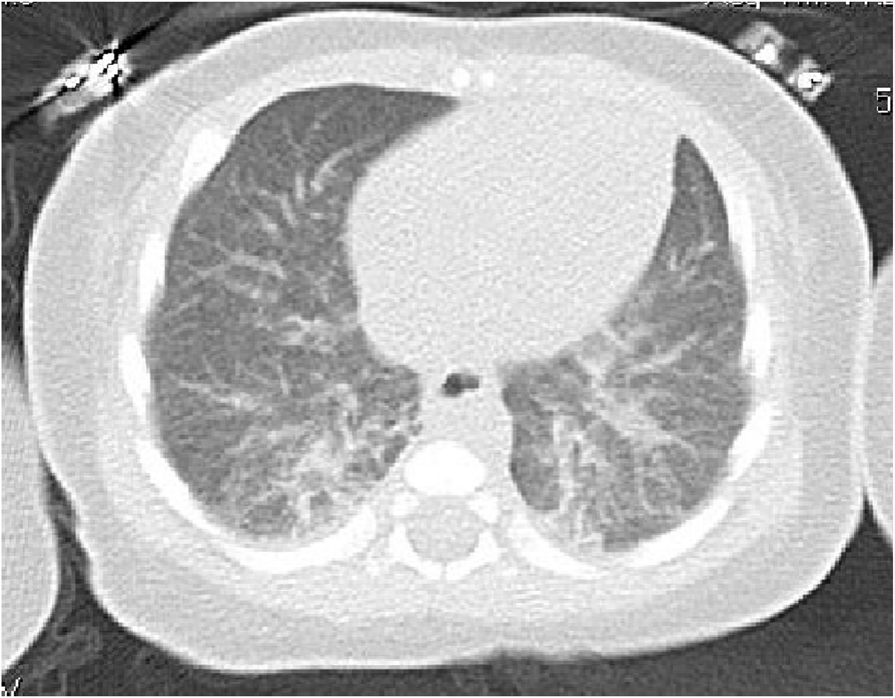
Chest CT demonstrated bilateral ground-glass infiltrates
